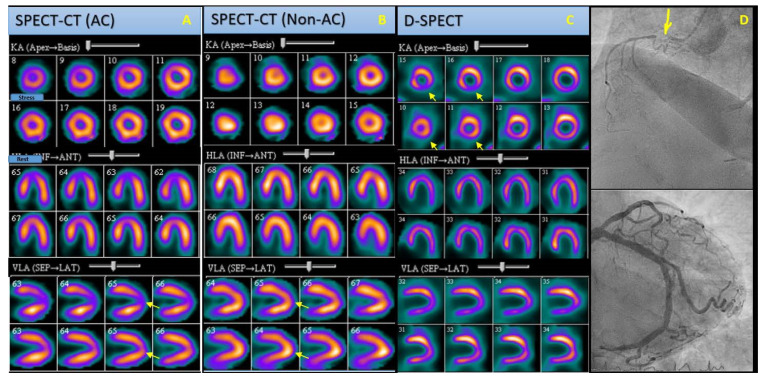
SPECT-CT and CZT-SPECT cardiac perfusion (stress: upper rows, rest: lower rows). Polar maps and angiography images of an obese 82-year-old woman with clinical presentation of stable angina and a medical history of hypertension. (A) Non-AC SPECT-CT images reveal reduced radiotracer uptake in the apical wall during the stress phase, which is reversed on rest phase images (arrows), indicating either an attenuation artifact or a reversible perfusion defect. (B) AC SPECT-CT images demonstrating a normal perfusion scan (arrows). (C) CZT-SPECT images present reduced activity in the inferior wall in stress phase, which is reperfused on rest phase images (arrows), indicative of reversible ischemia in the inferior wall. (D) Angiography images of the same patient demonstrating presence of stenosis in RCA (arrow), which correlates perfectly with the CZT-SPECT results. *SPECT-CT: Single Photon Emission Computed Tomography-Computed Tomography; CZT-SPECT: Digital Single Photon Emission Computed Tomography; Non-AC: non-Attenuation Corrected; AC: Attenuation Corrected, RSA: Right Coronary Artery*.
